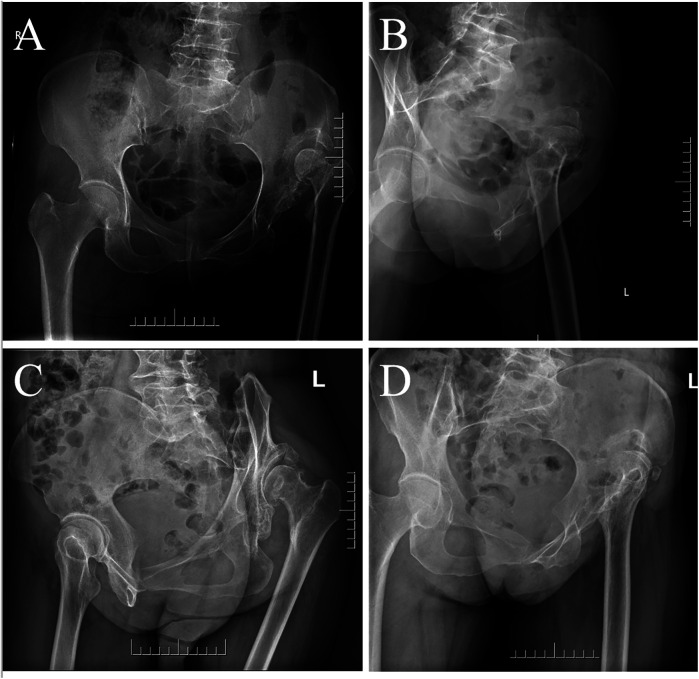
Long-term unreduced posterior hip dislocation in different X-ray projection postures. (A) Anteroposterior radiograph of the pelvis. (B) Lateral radiograph of the femur. (C) Obturator oblique radiograph of the pelvis. (D) Ilium oblique radiograph of the pelvis.
